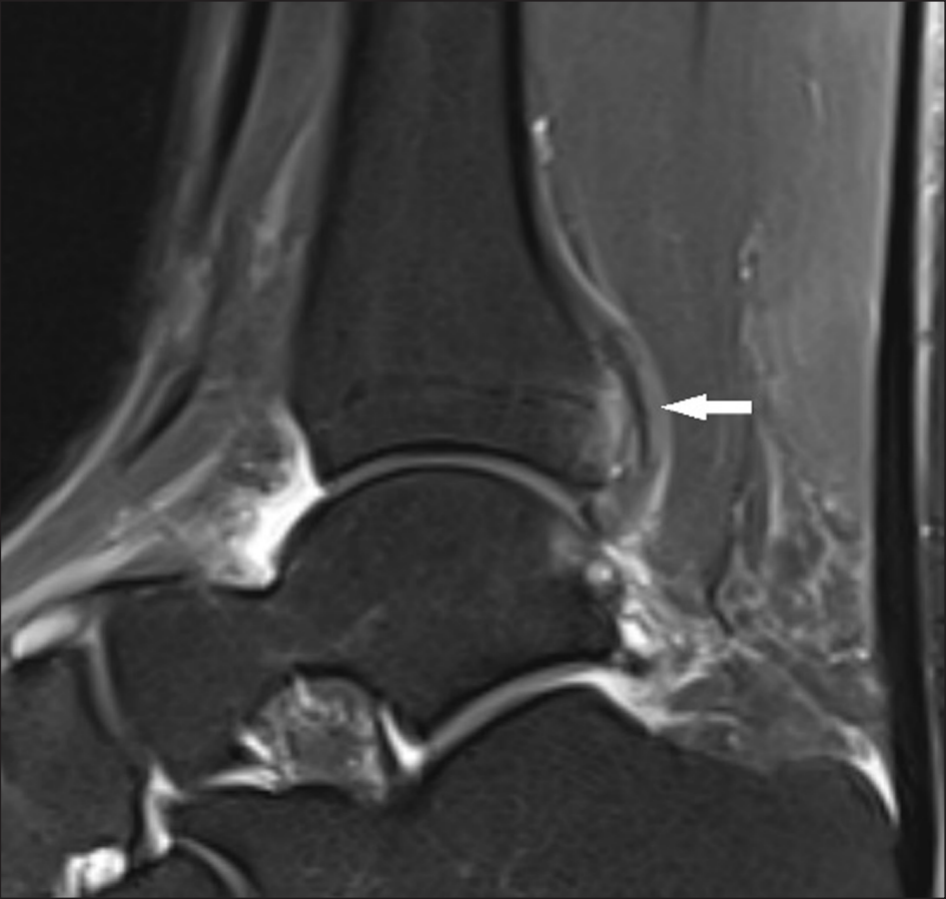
Subsequent CT-examination confirmed the diagnosis of a PTLF with curvilinear morphology (Figure 3).
